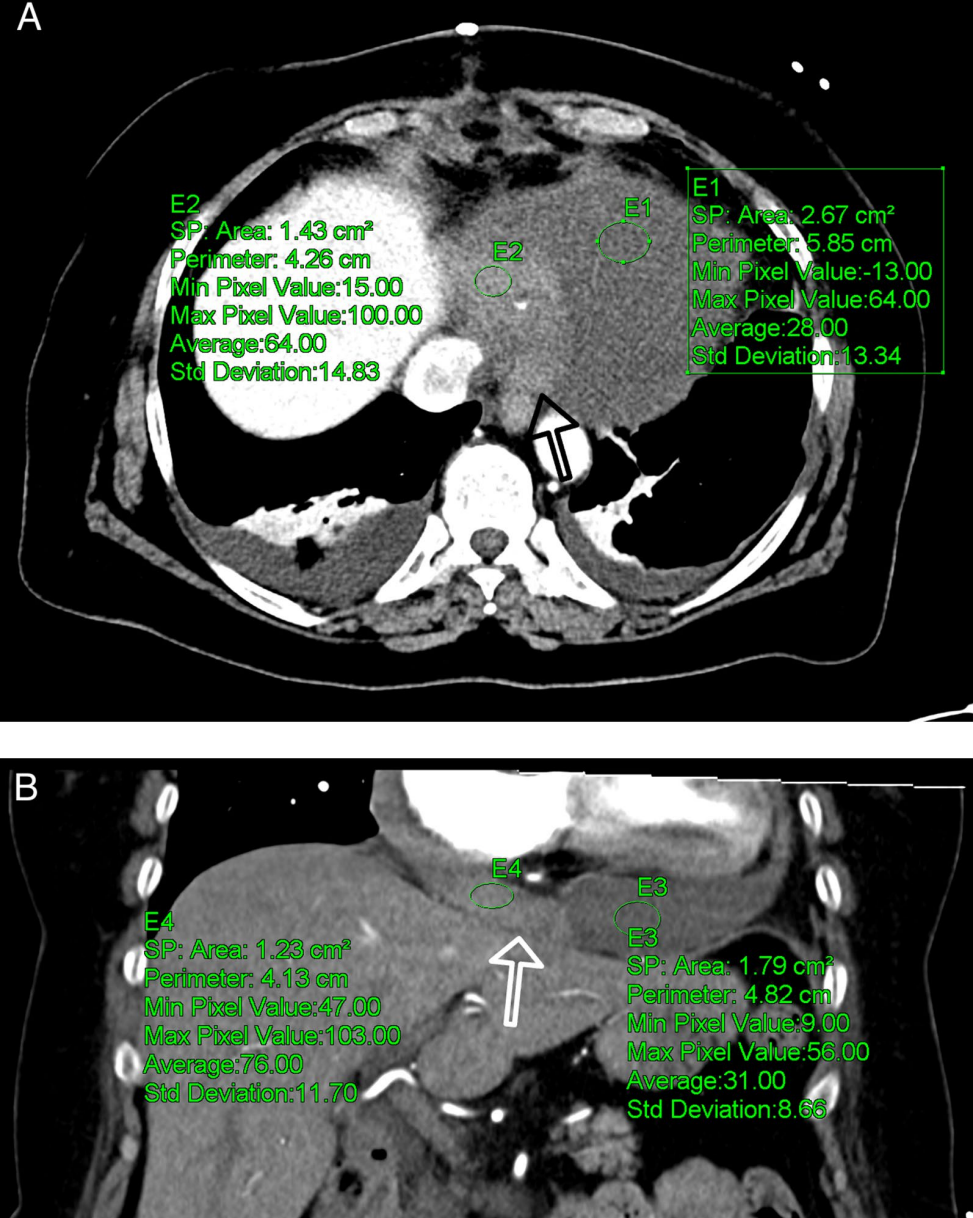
Abdominal CT-scan in axial (A) and coronal (B) view with contrast medium in portal-venous phase shows increased density (64–76 Hounsfield units) of the fluid in the pericardium (normal fluid 28–31 Hounsfield units), which is compatible with acute hemopericardium ( min.: minimum; max.: maximum; Std. deviation: standard deviation)
